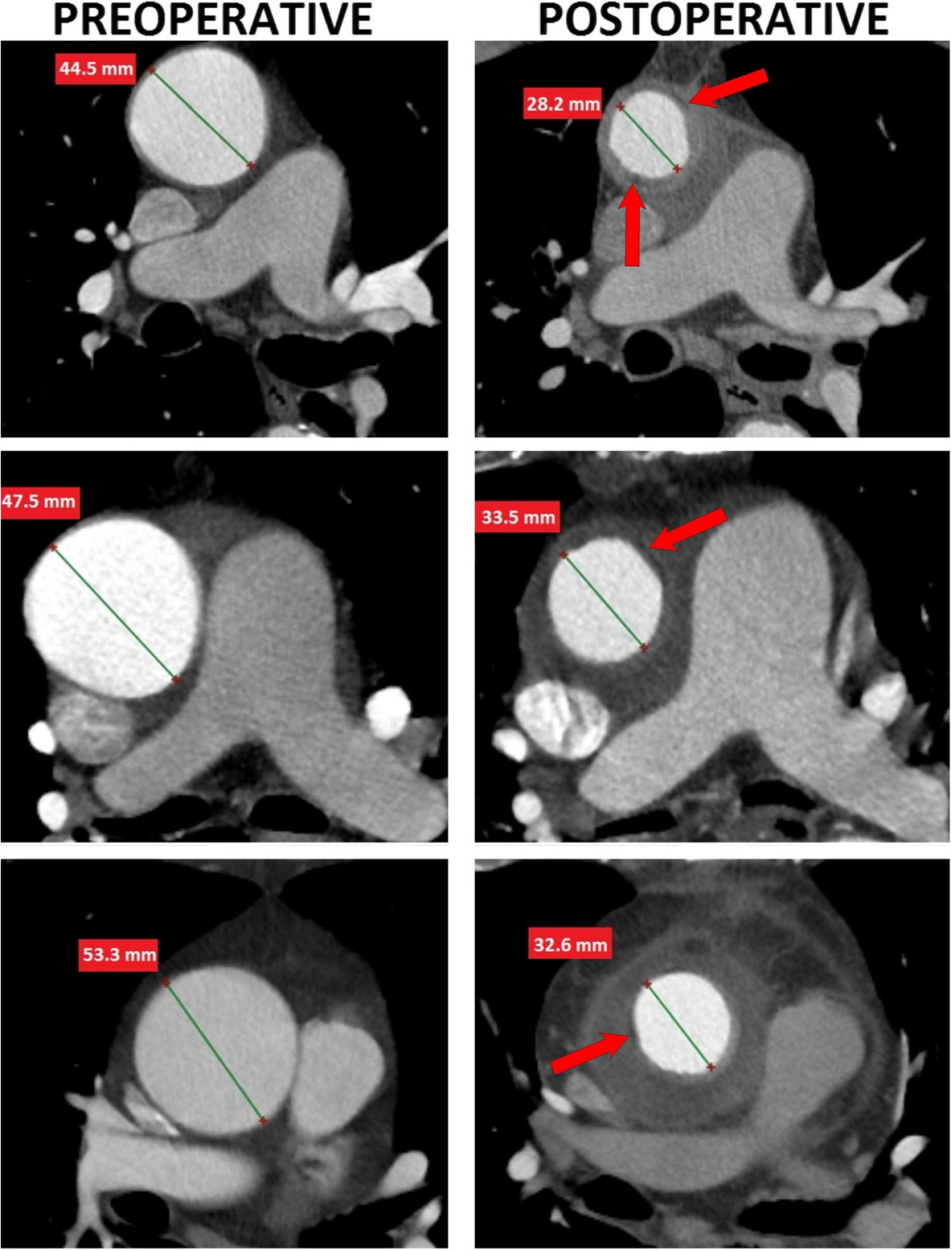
Preoperative and postoperative angio-CT images of patients who underwent the wrapping procedures. Red arrows point toward the uncontrasted layer between the vascular prosthesis and the aortic wall
